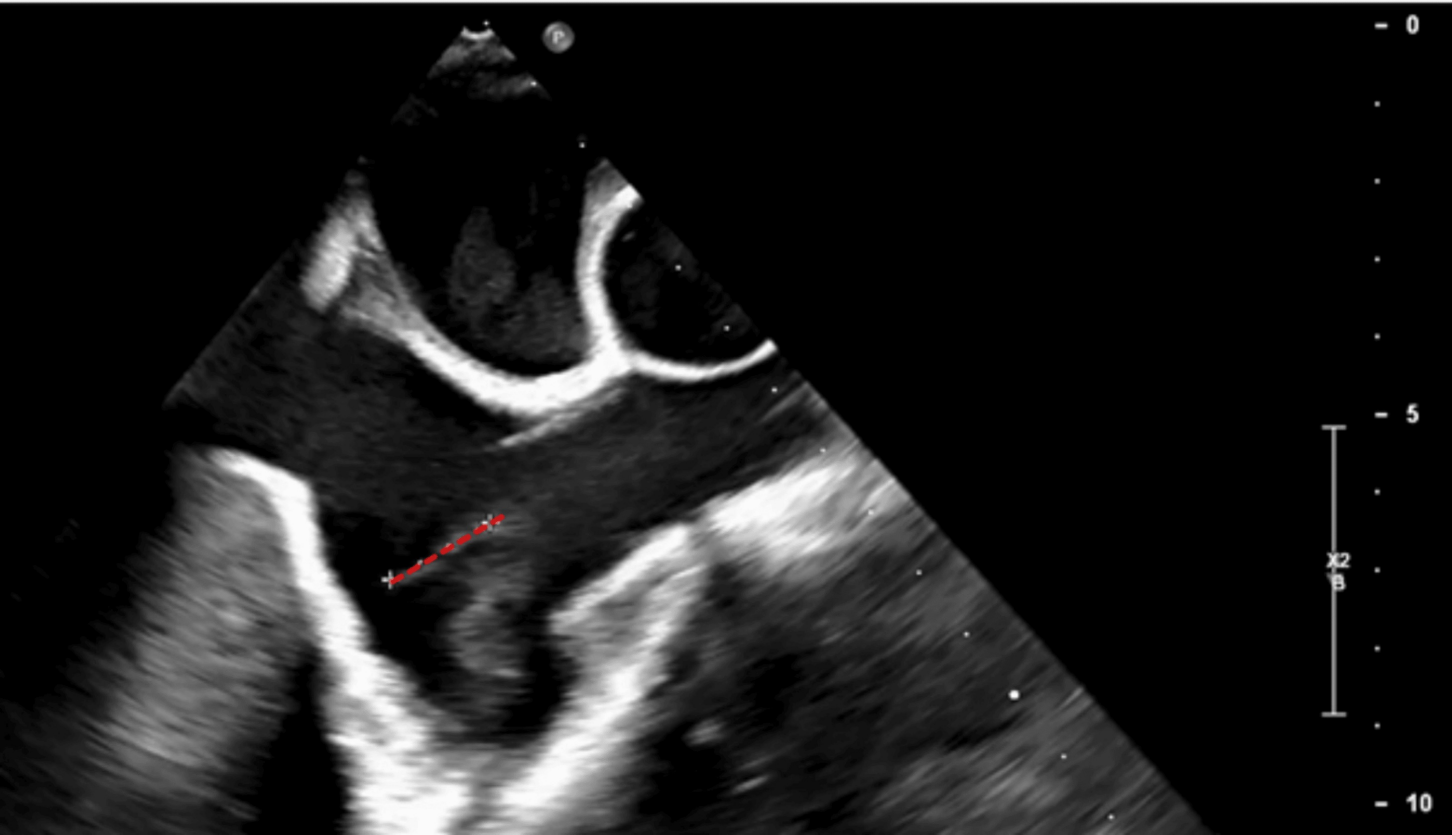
Mid-position transoesophageal echocardiography image A mass measuring approximately 15 millimeters can be seen attached to one of the pacemaker leads in the right atrium (red dotted line in the image, representing the measurement).
